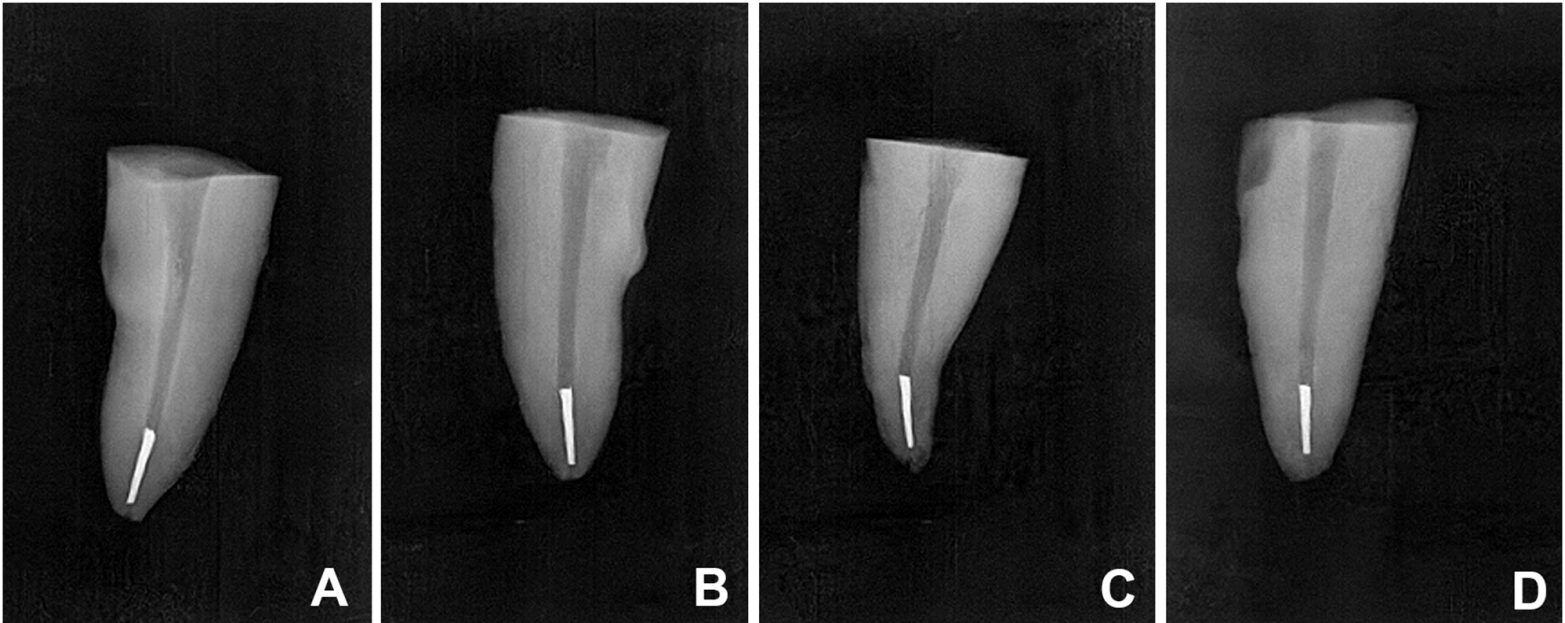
Radiographic images of approximately 3 mm apically file fragment
